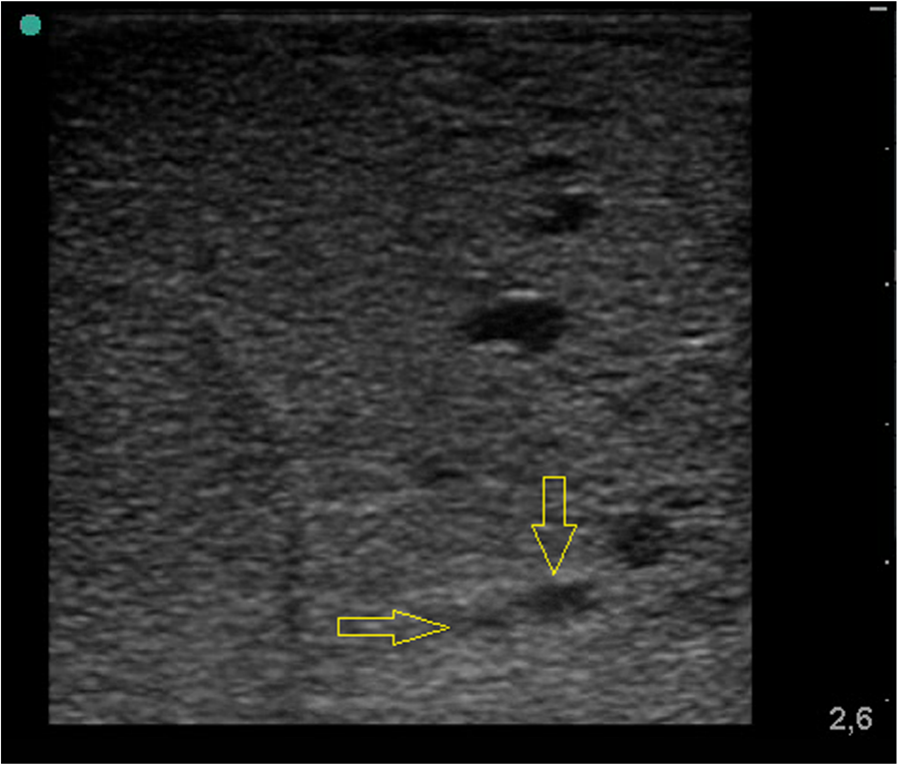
Sonographic image after an erosive cavitation event in flooded lung; *arrow* indicates the focal zone with echoless structure
